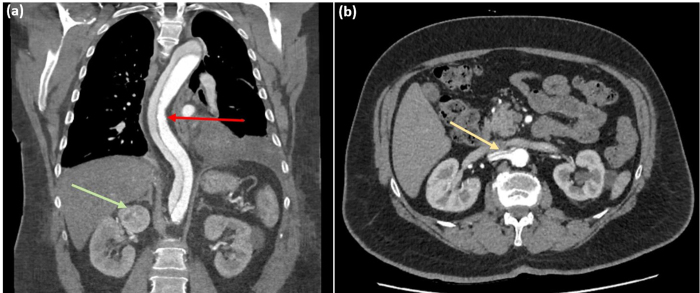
(a) Arterial phase computed tomography scan of chest and upper abdomen demonstrating a type A aortic dissection (red arrow). The dissection extends from below the aortic root to below the level of the renal arteries. A 47×37×46mm mass lesion, highly suspicious of a renal cell carcinoma is incidentally found on the upper pole of the right kidney (green arrow). (b) The true and false lumens of the aortic dissection extend into the right renal artery (yellow arrow).
